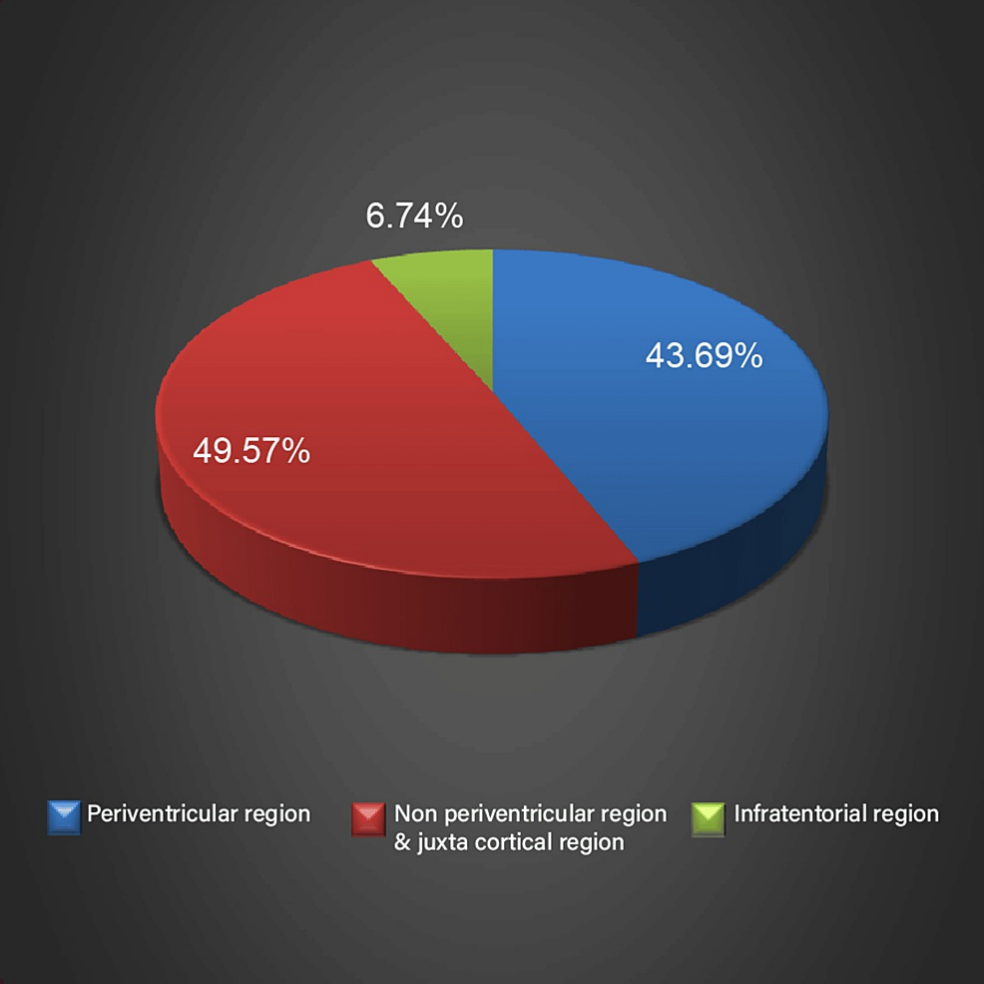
Distribution of lesions on 3D-FLAIR
